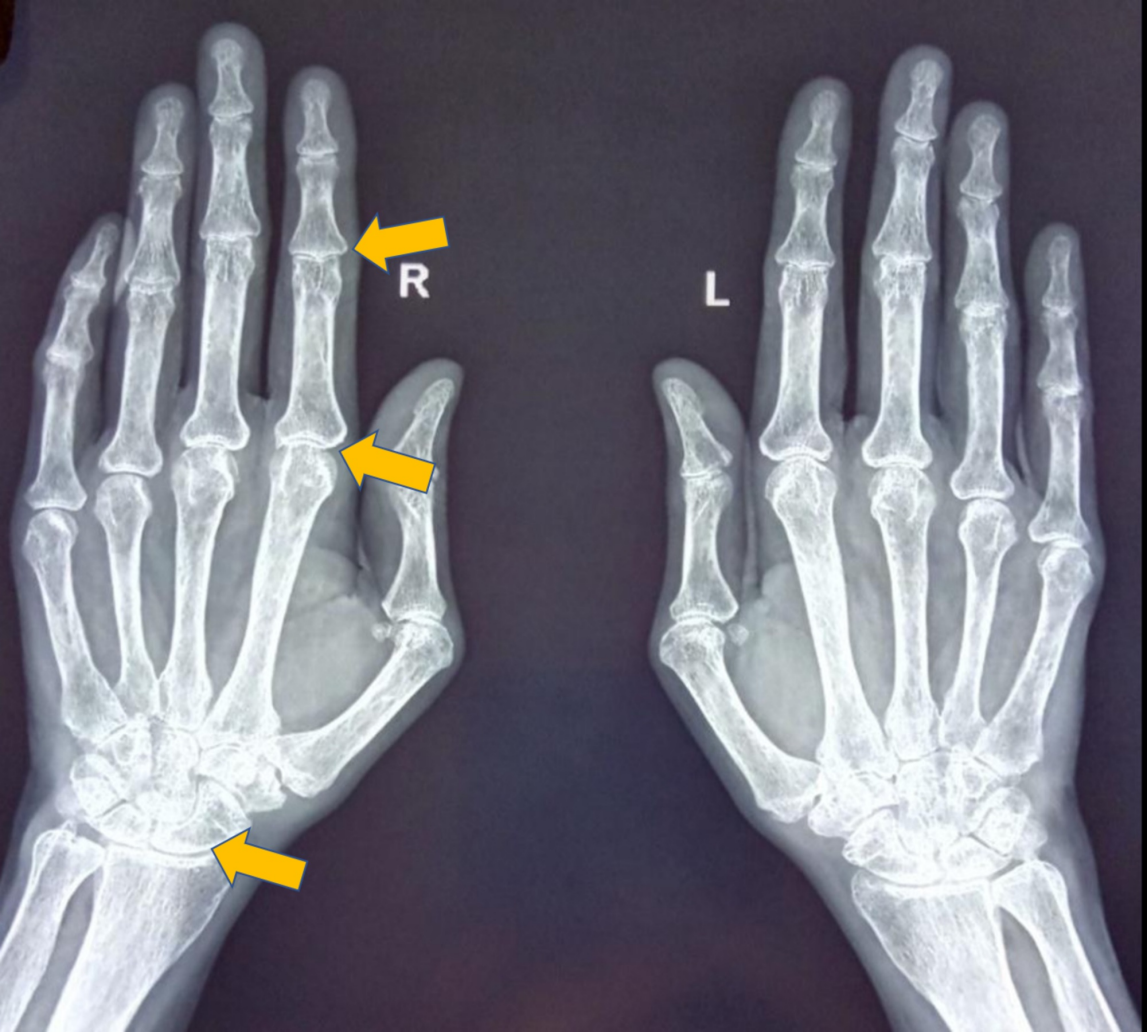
X-ray of both hands revealed subchondral erosion of the wrist, PIP, and MCP joints along with narrowing of these joint spaces with juxtaarticular osteopenia PIP: proximal interphalangeal; MCP: metacarpophalangeal.
